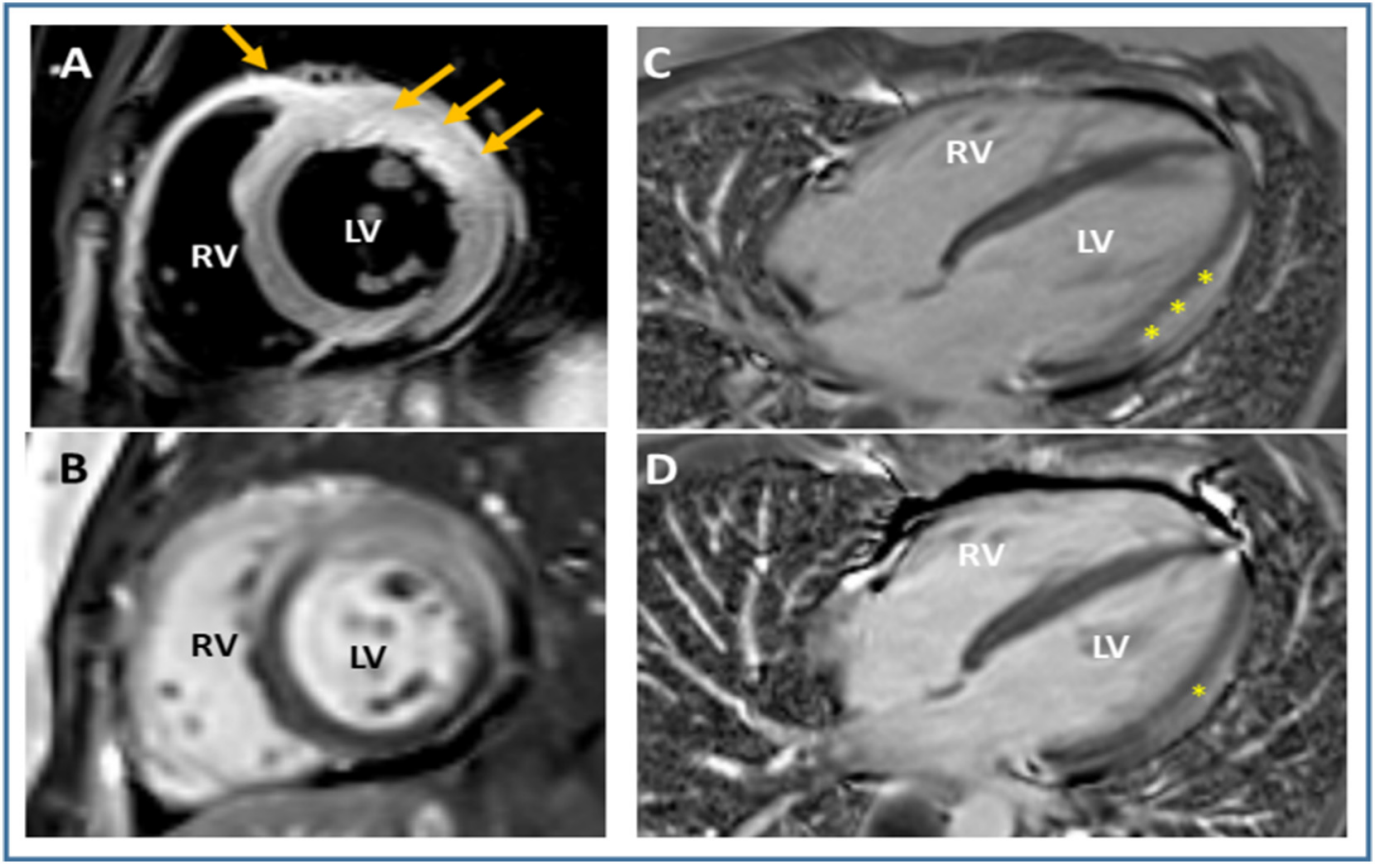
Initial CMR (A-C) and follow-up CMR at 3 months (D). Short-axis T2-weighted short-tau inversion recovery view (A) shows severe edema (arrows) in the anteroseptal, anterior, and anterolateral wall of the left ventricle (LV) as well in the anterior free wall of the right ventricle (RV). In addition, the pericardium covering the affected myocardium shows increased signal and thickening. Distribution of LGE (B) corresponds well with extent of edema, and these changes appear mainly transmurally across the anterior wall. In the 4-chamber-view, LGE (asterisks) is initially (C) extensively distributed in the midmyocardial and subepicardial layers of the LV wall and in the pericardium covering the lesion. At follow-up CMR (D) the presence of LGE is considerably reduced. CMR, cardiac magnetic resonance imaging; LGE, late gadolinium enhancement.
